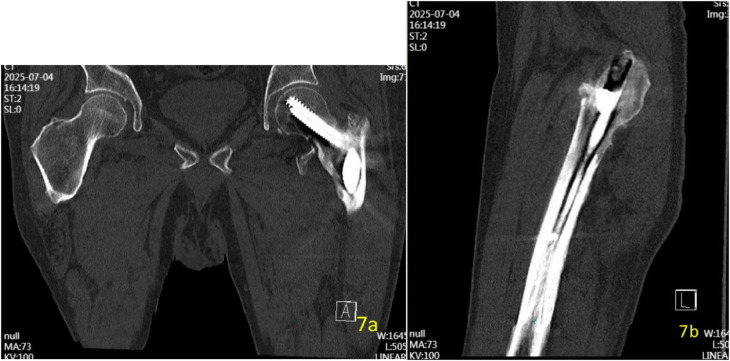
Two-panel CT scan series at the 5-month follow-up. The left image (a, coronal reconstruction) and the right image (b, sagittal reconstruction) demonstrate complete bony union of the intertrochanteric fracture.
